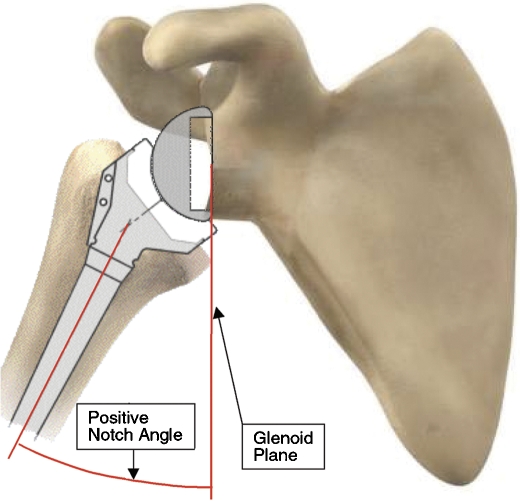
Delta CTA prosthesis build in the average anatomical model based on 200 measured scapulae, with specific interest in the shape of the glenoid cavity, the infraglenoid tubercle, and the lateral border of the scapula. The prosthesis has a flat baseplate situated as distally as possible to ensure full bony coverage of this baseplate with the inferior screw surrounded by minimum 2 mm bone, and a glenosphere 36 mm with a standard polyethylene cup. The notch angand le is the maximum adduction angle before a conflict arises between the PE cup and scapular pillar.
